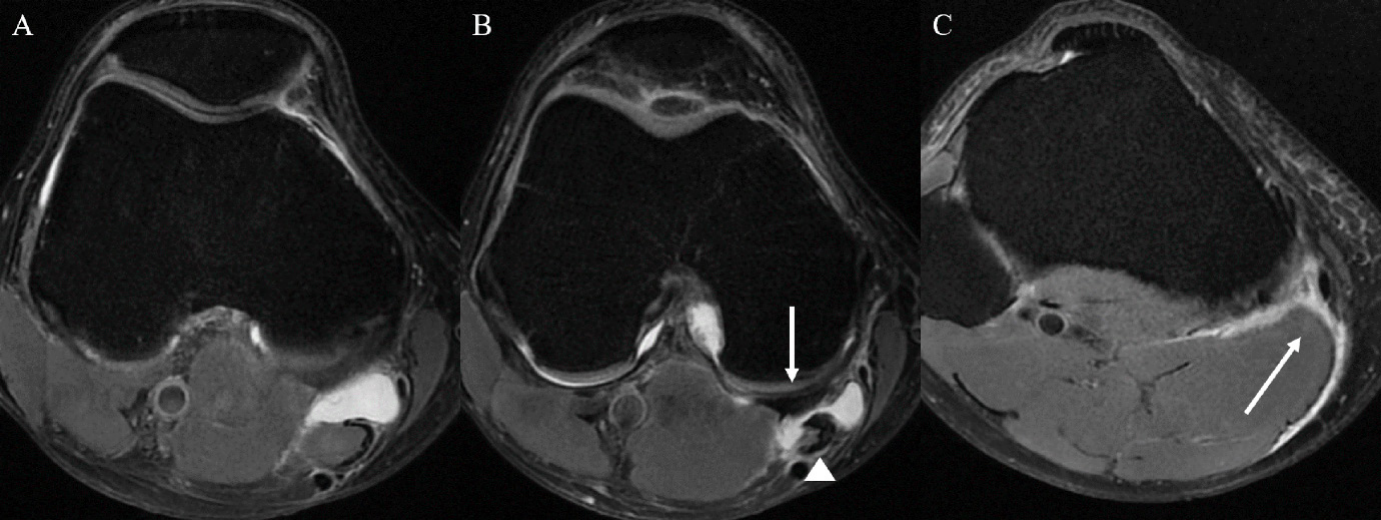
Right knee MRI in a 48-year-old male patient with Baker’s cyst rupture. (A, B) On axial T2 image, there is a cyst between the semimembranosus tendon (arrow) and medial head of gastrocnemius tendon (arrowhead) (C) with fluid dispersion (arrow) along intermuscular fat plane.
